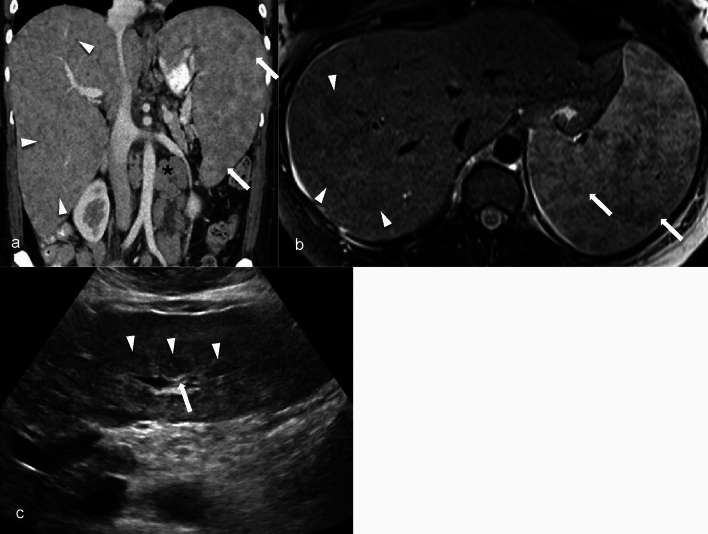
39-year-old female with a history of sarcoidosis and cholestatic hepatitis. Coronal contrast enhanced CT demonstrates enlarged liver and spleen with heterogeneous appearing parenchyma secondary to multiple hypodense nodules scattered throughout the liver (arrowheads, a–b) and spleen (arrows, a–b). Multiple enlarged retroperitoneal lymph nodes are present (asterix, a). Axial T2W MR image shows hypointense nodules scattered throughout the liver (arrowheads, b) and spleen parenchyma (arrows, b). Ultrasound images demonstrate multiple hypoechoic nodules in the liver (arrowheads, c) with mass effect on the portal triads (arrow, c) from non-caseating granulomas
